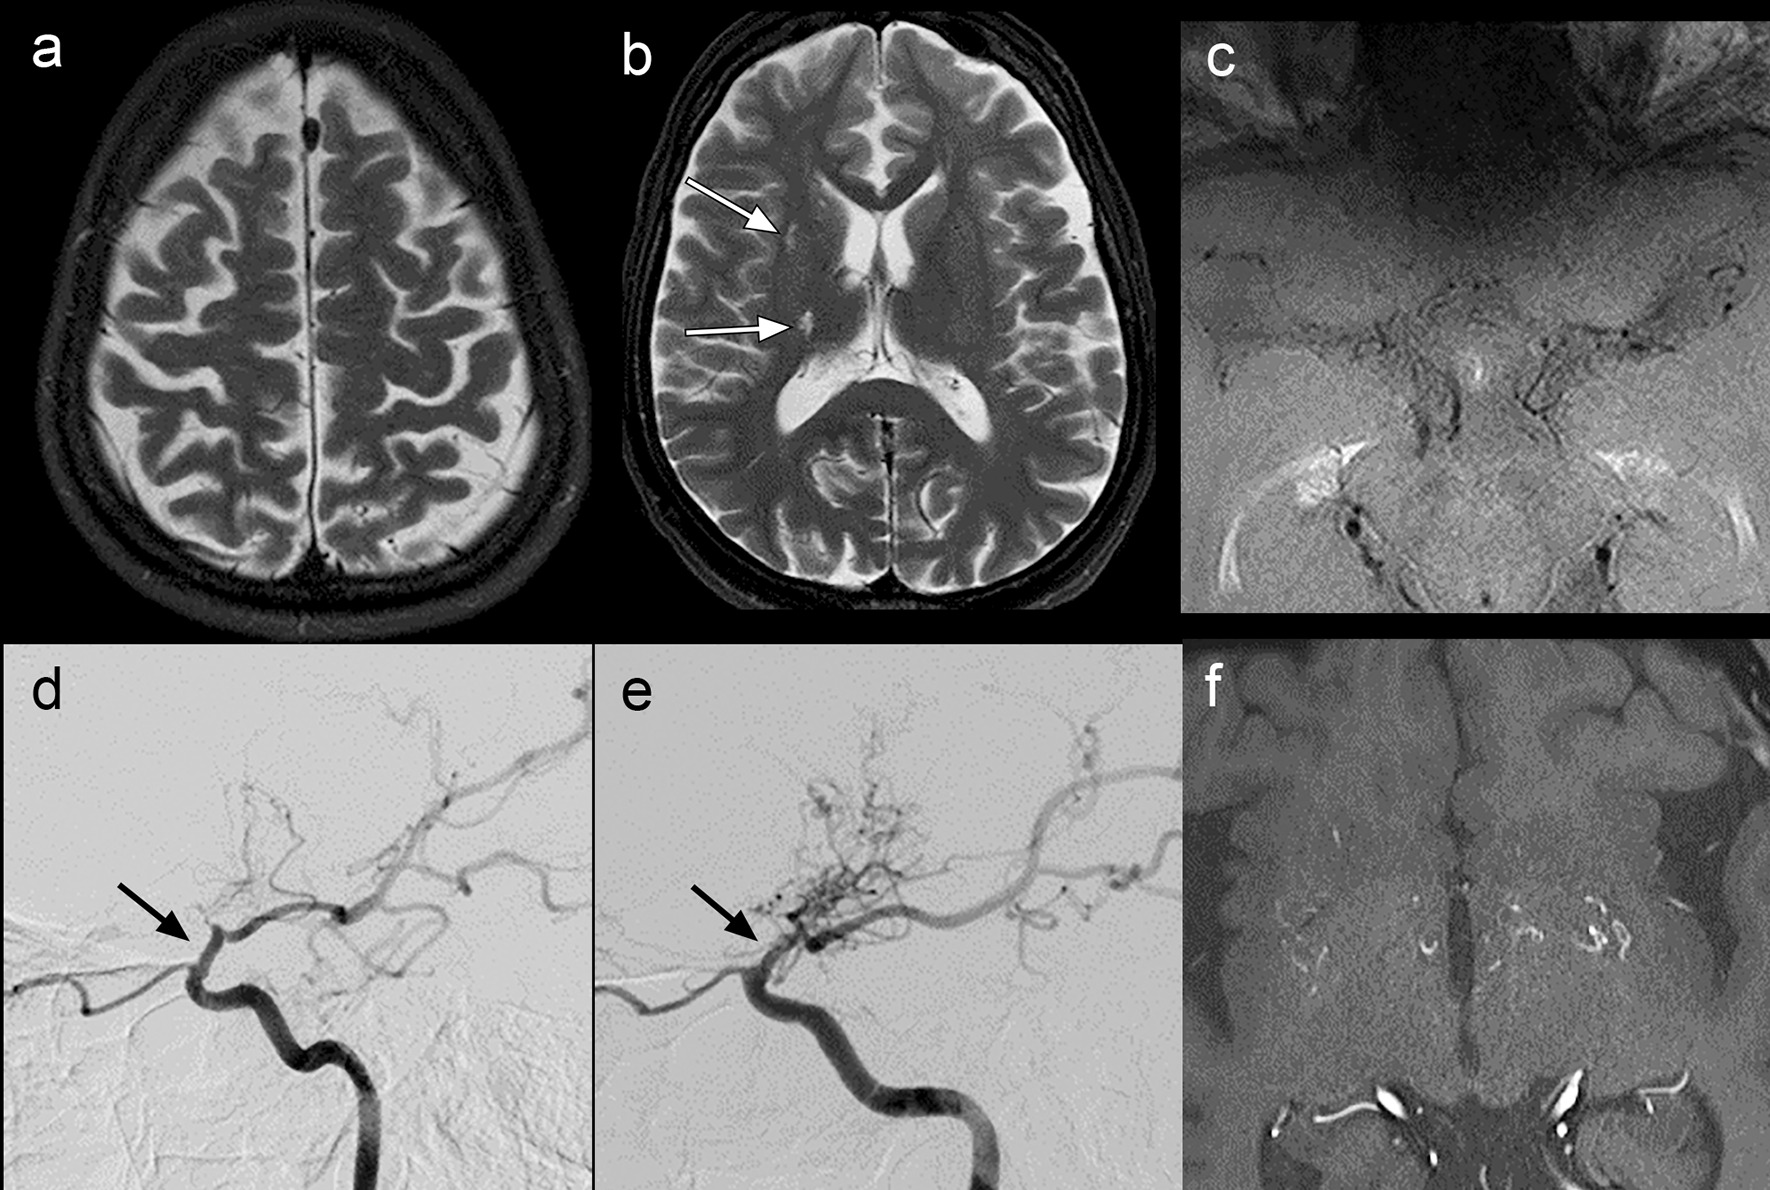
Example of a completely obliterated arterial appearance on VWI. A 44-year-old female with a 2-year history of paresthesia and clinical findings compatible with right hemispheric infarcts had no vascular risk factors, an unremarkable work-up for rheumatologic conditions or other causes of a Moyamoya syndrome. MRI head, including axial FSE T2 weighted images (a, b) demonstrate greater than expected generalized volume loss for age in the bilateral cerebral hemispheres and chronic lacunar infarcts (white arrow, b) in the right internal capsule, subinsular white matter (white arrow, b), coronal radiata (not shown), and caudate head (not shown). Axial VWI with contrast (c) demonstrates complete non-visualization of M1 segment arteries in the expected MCA cistern regions (on this and all other adjacent images), compatible with a VWI appearance of complete obliteration. Right lateral DSA (d) and left lateral DSA (e) show expected tapering of the terminal ICAs bilaterally (black arrows) and proliferation of moyamoya type collateral arteries, greater on the left. Axial 3D-TOF MRA again shows the moyamoya collaterals in both basal ganglia (f)
